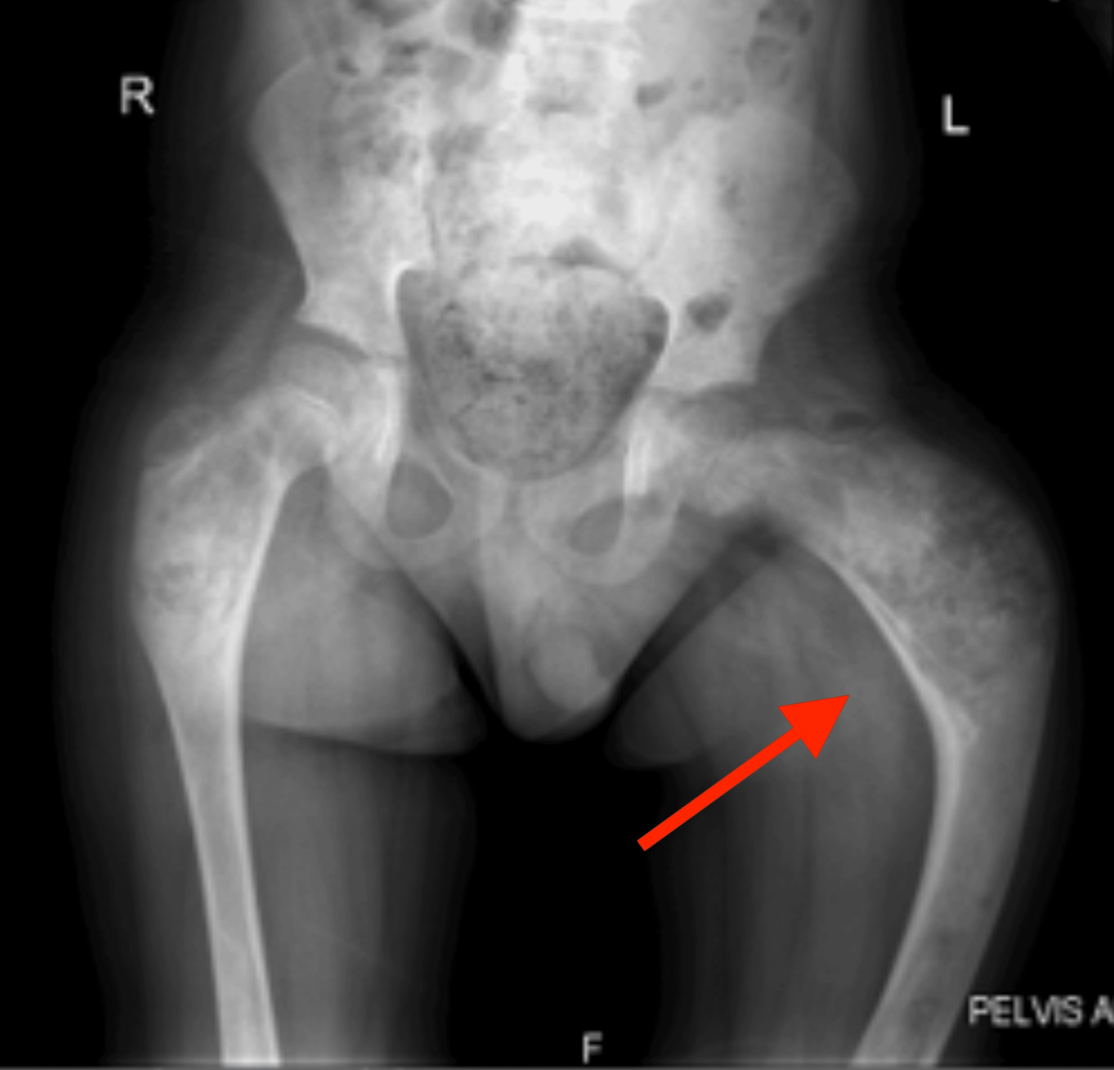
X-ray of the hip showing Shepherd's crook deformity
